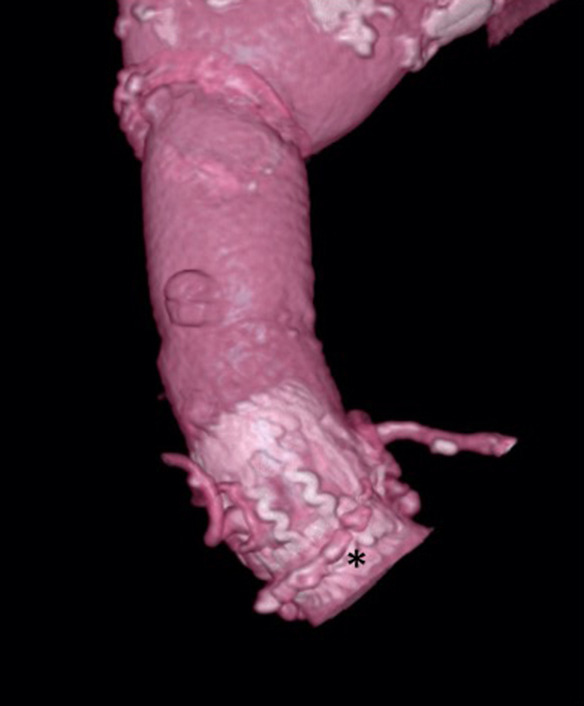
Postoperative CT image of SAV and aortic root. ∗Previous surgery pledgets.
